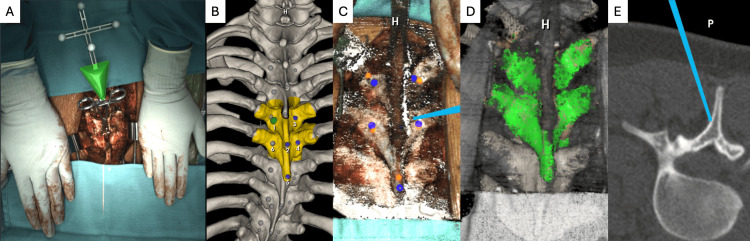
(A) Intraoperative view of an exposed thoracic spine as seen by the machine vision system, with the flex array highlighted in green, indicating appropriate device registration. Surgical towels placed cranially and caudally cover other exposed spinal segments, while the surgeon’s hands cover reflective surgical retractors to optimize device registration. (B) The 3D reconstruction of a patient’s preoperative CT scan with T5 and T6 highlighted for device registration using machine vision navigation. Numbered dots are used to mark specific bony landmarks, which are then selected by the surgeon using a navigated probe. (C) A navigated probe (blue line) is used to select landmarks on the patient (orange dots), which are then correlated to the landmarks identified on the CT scan (blue dots). (D) Device registration of the intraoperative spinal topography overlaid onto the preoperative CT scan. (E) Projection of the navigated probe marking a thoracic lamina onto the intraoperative CT scan. CT: Computed tomography
